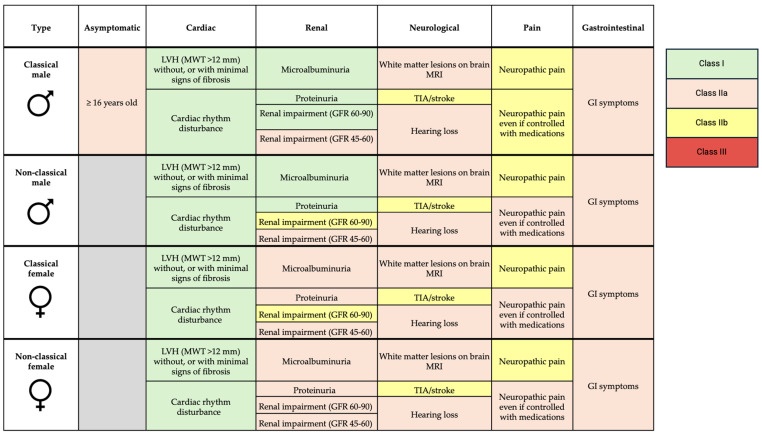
Indications for enzyme replacement therapy in FD. LVH—left ventricular hypertrophy; MWT—maximal wall thickness; GFR—glomerular filtration rate; MRI—magnetic resonance imaging; TIA—transient ischemic attack; GI—gastrointestinal.
